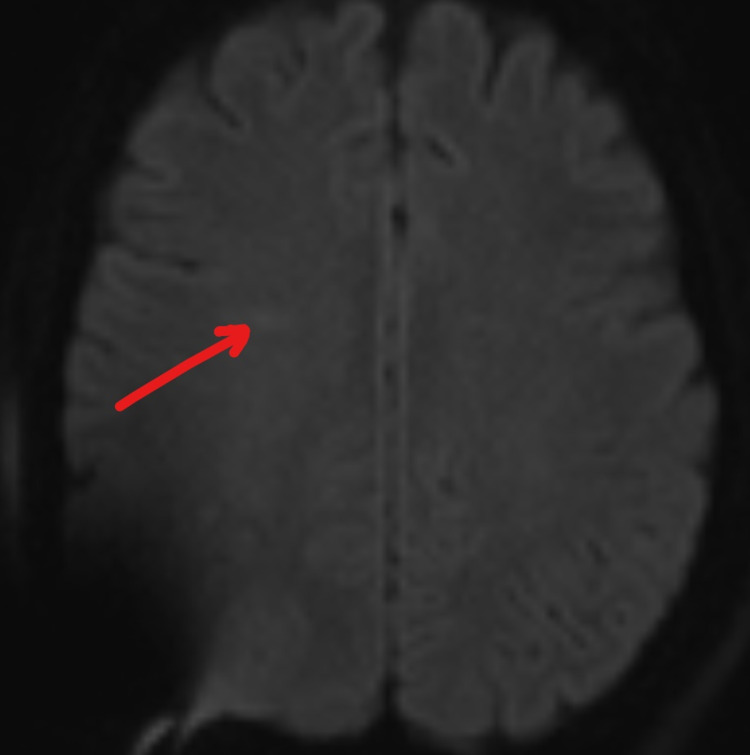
DWI of head MRI, Day 27. DWI: diffusion-weighted imaging.
